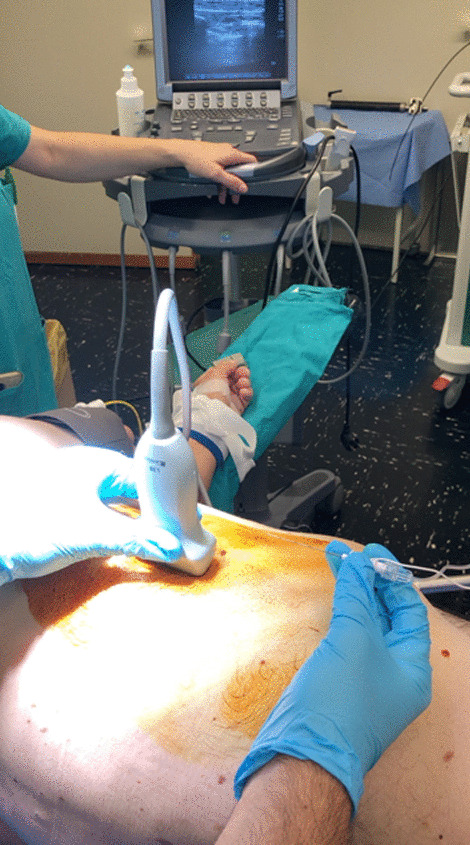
Ultrasonographic evaluation in serratus plane block (SAP block) during uniportal video-assisted thoracoscopy (u-VATS) surgery
